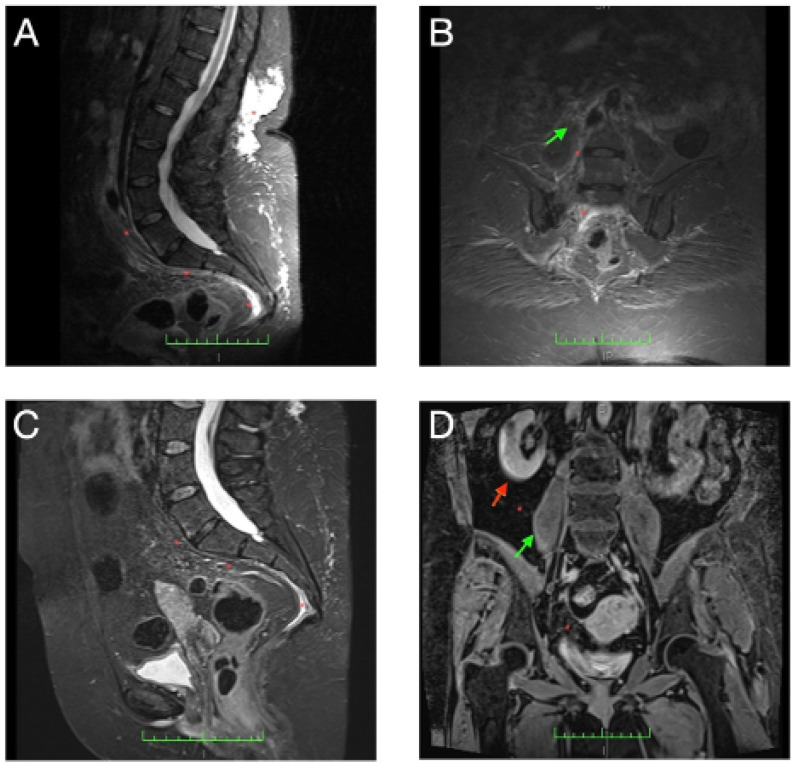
MRI of the lumbosacral spine and pelvis [February (A–B) and April 2019 (C–D)]. (A) February 2019-Sagittal T1-weighted fat-suppressed post-contrast MRI of the lumbosacral spine, demonstrating irregular enhancement extending from the lower lumbar levels into the right retroperitoneal and pelvic compartments (red asterisks). Additionally, a focal area of contrast enhancement is visible in the posterior paraspinal musculature at the mid-lumbar level, potentially reflecting spread of inflammation or secondary involvement of adjacent soft tissues. (B) February 2019-Coronal T1-weighted fat-suppressed post contrast MRI demonstrating asymmetric enhancement in the right retroperitoneum, with the red asterisks denoting regions of increased post-contrast signal. The green arrow highlights the adjacent right psoas muscle. Subtle surrounding fat stranding is also noted, consistent with an underlying inflammatory or infiltrative process. (C) April 2019-Sagittal T2-weighted MRI demonstrating hyperintense signal in the presacral space and anterior to the sacrum, consistent with soft tissue oedema and/or inflammation. Notably, there is substantial resolution of the previously noted retroperitoneal inflammatory changes. (D) April 2019-Coronal T1-weighted fat-suppressed post-contrast image reveals asymmetric soft tissue thickening and enhancement in the right retroperitoneal region. Red asterisks denote regions of retroperitoneal fat stranding and subtle soft tissue enhancement. The green arrow points to the right psoas muscle, which shows abnormal enhancement. The red arrow highlights perinephric fat stranding surrounding the right kidney.
